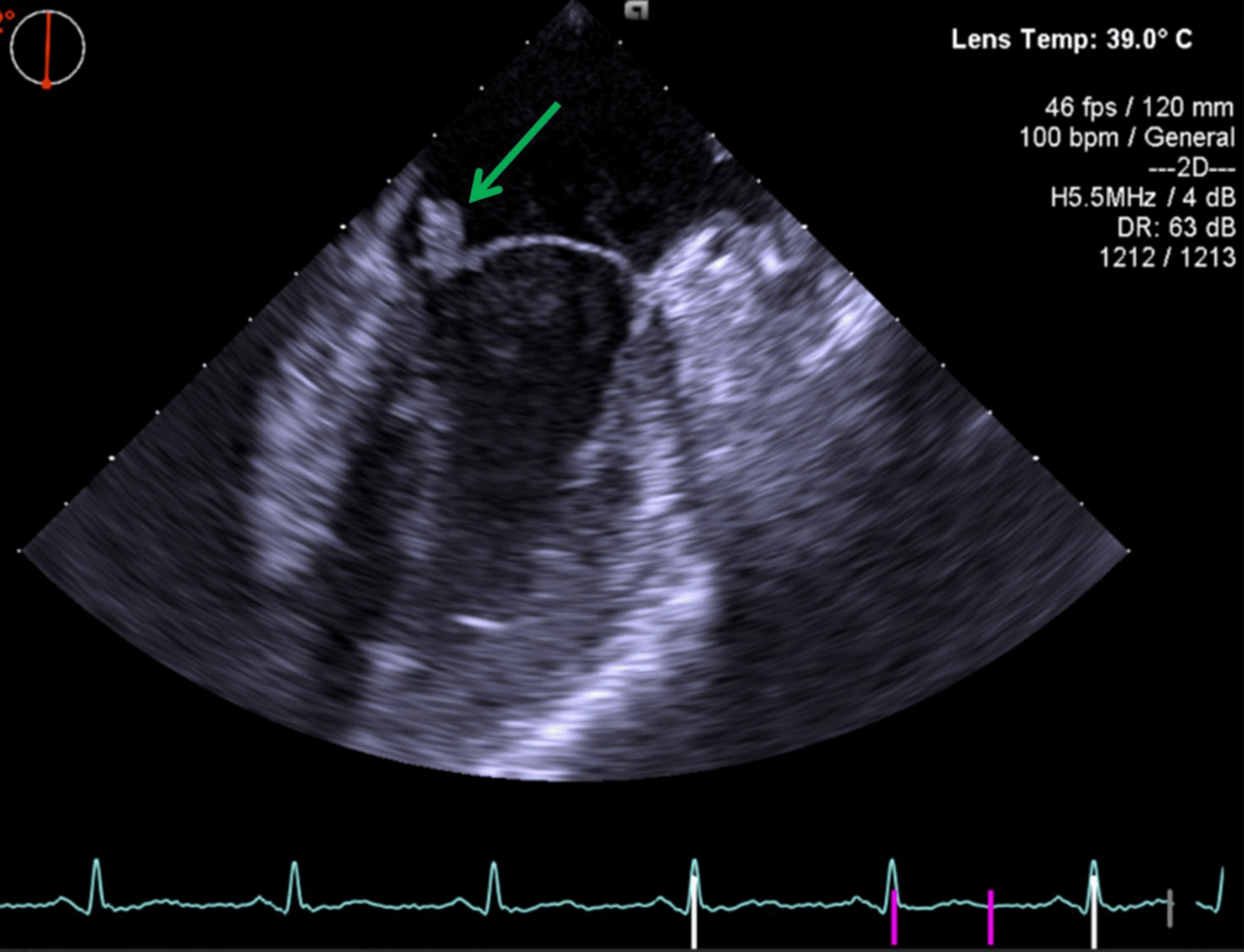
Vegetation on the leaflet of the mitral valve in transesophageal echocardiogram
